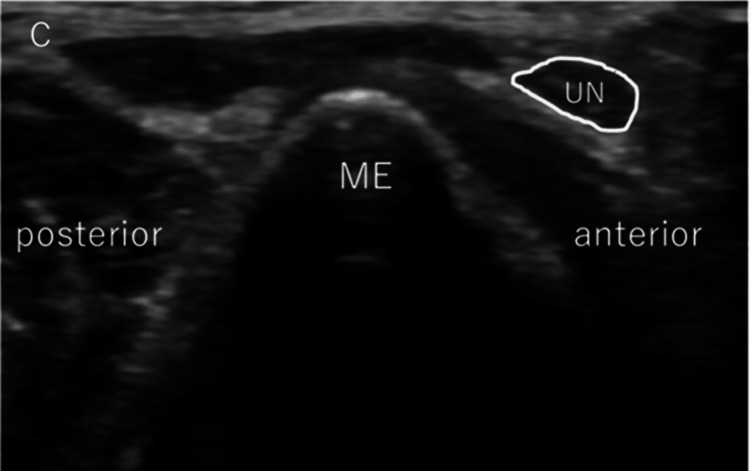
Assessment of ulnar nerve instability: dislocation type Transverse US images of the ulnar nerve show dislocation. ME: medial epicondyle; UN: ulnar nerve; US: ultrasonography
